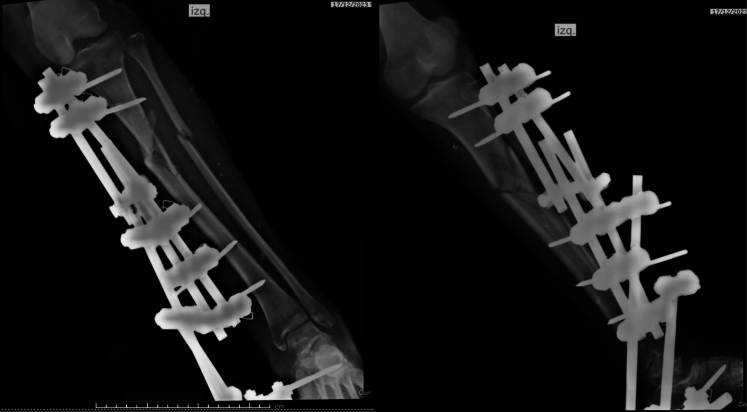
Anteroposterior (AP) and lateral x-ray of the left leg showing external fixator and satisfactory alignment.
